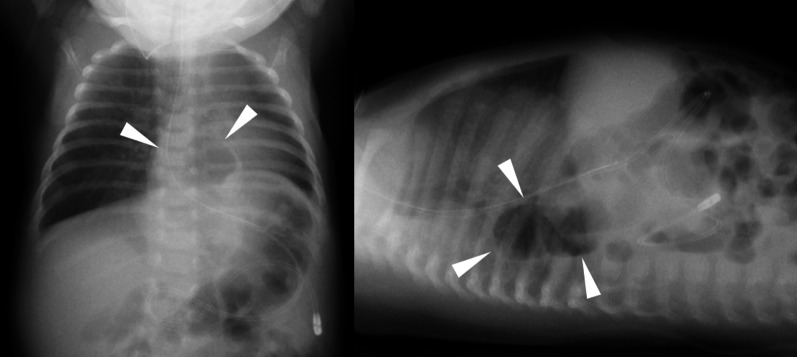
Chest radiograph of a suspected recurrent left diaphragmatic hernia. Chest radiograph on postoperative day 35 shows bowel gas is present in the thoracic cavity (arrowhead)
